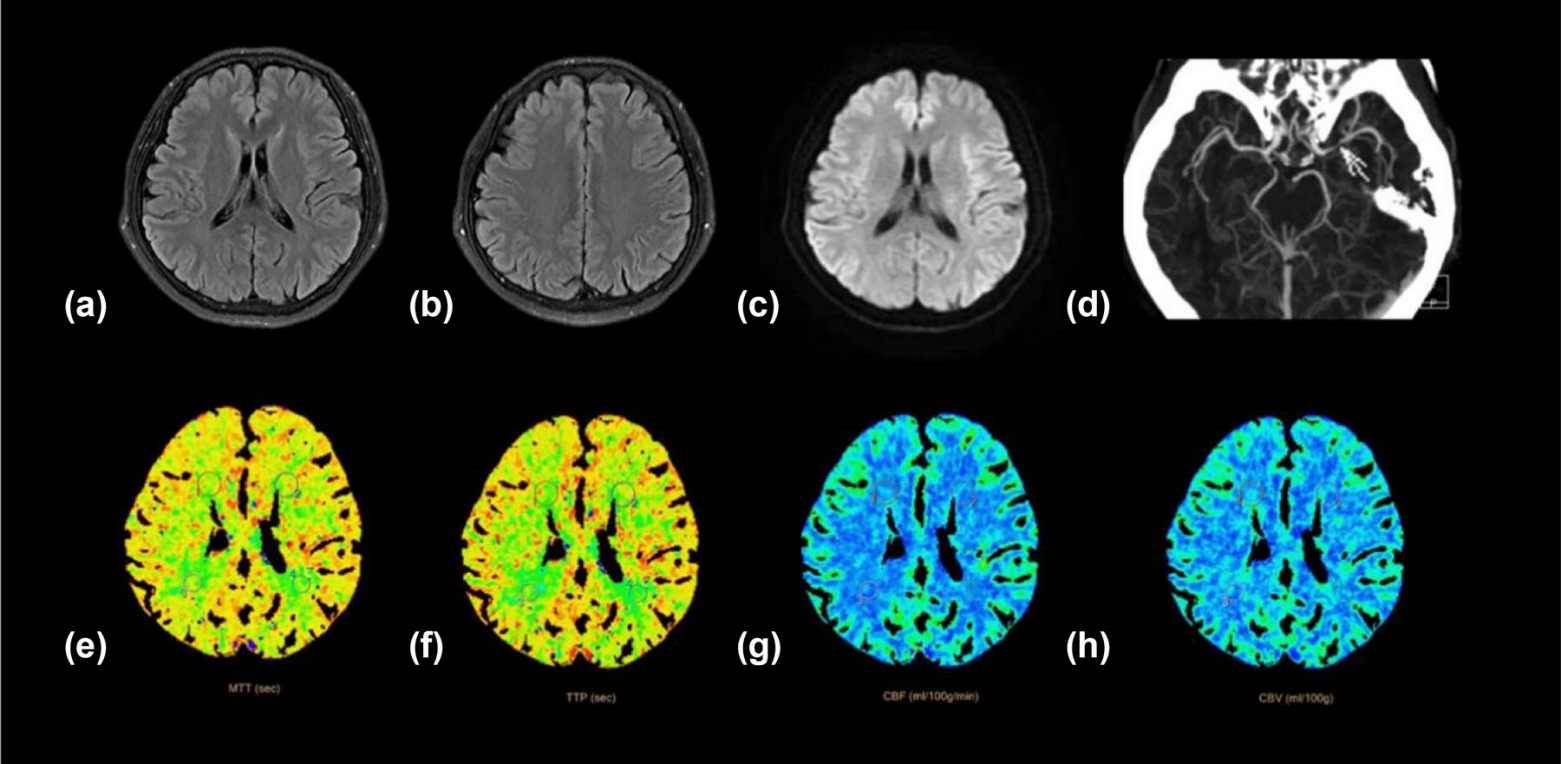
Another patient with severe stenosis of the left middle cerebral artery (D), and normal cerebral perfusion (E, H).There was little difference in WMHs between the two sides (A, B); DWI did not show abnormalities (C).
